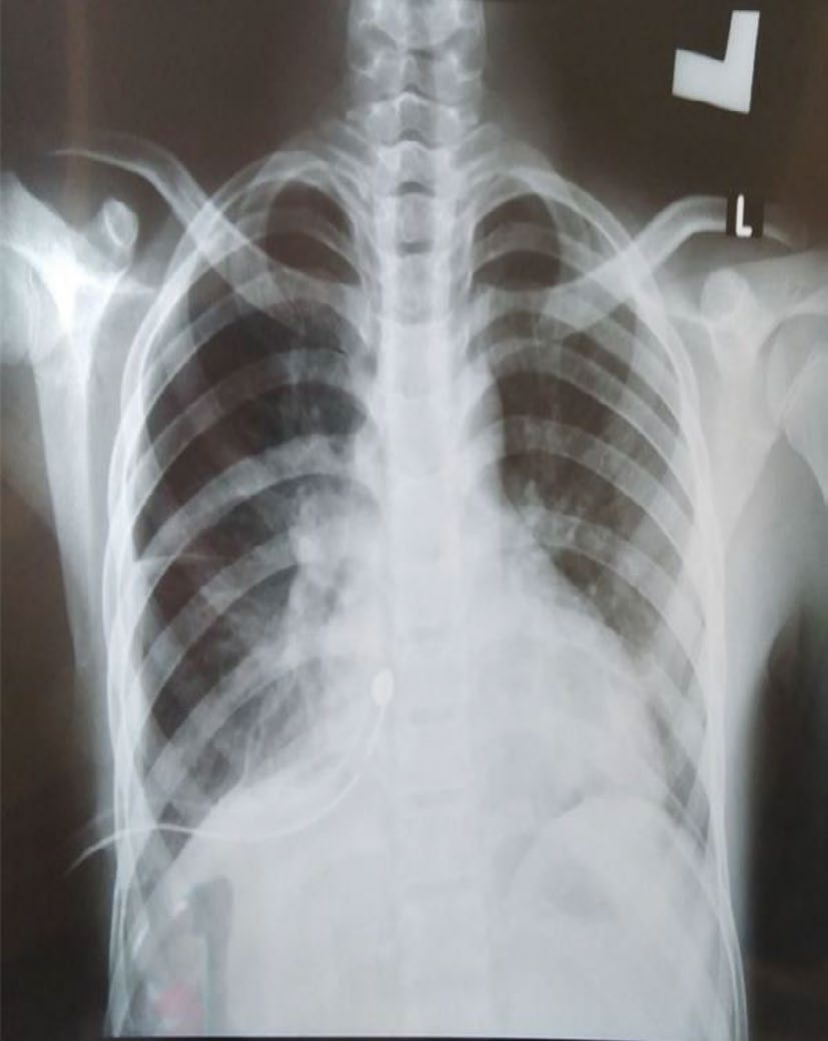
Poster‐anterior chest radiograph at discharge (after the insertion of chest tube)
